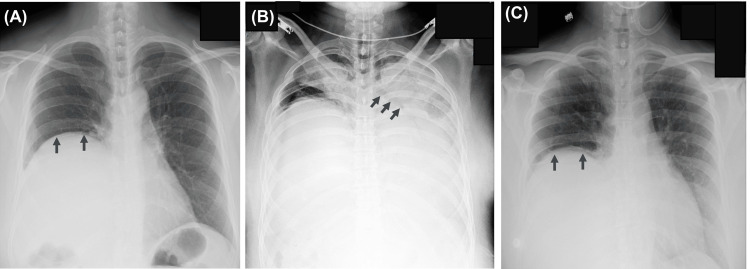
Chest X-ray during perioperative period (A) Preoperative chest X-ray showed elevation of the right hemidiaphragm, suggesting possible phrenic nerve paralysis. No obvious abnormality was observed in the lungs. (B) A chest X-ray obtained immediately postoperatively showed bilateral infiltrates with air bronchograms, suggesting possible pulmonary edema. (C) A chest X-ray obtained on postoperative day 1 showed that the infiltrates in the lung fields had largely resolved, although they persisted in the left lower lung field. Arrows in (A) and (C) indicate elevation of the right hemidiaphragm. Arrows in (B) indicate an air bronchogram.
